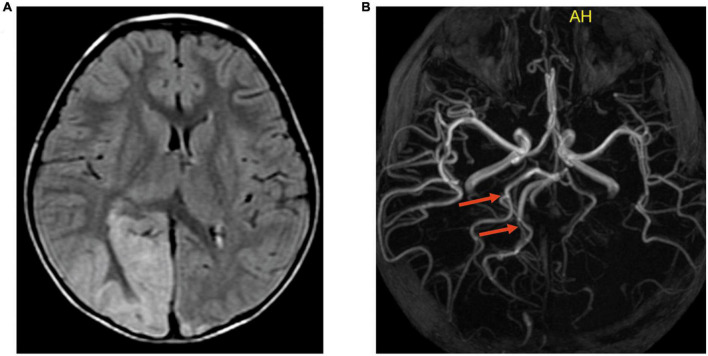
Slightly dilated cerebral artery and the branches relevant to the lesion on MRA. Case 9, A 5-year-old boy with MELAS. (A) Transverse FLAIR images showed cortical swelling in the right occipital lobe. (B) MRA showed that the right posterior cerebral artery and the branches relevant to the lesion (indicated by the red arrows) were slightly dilated compared with the left.
